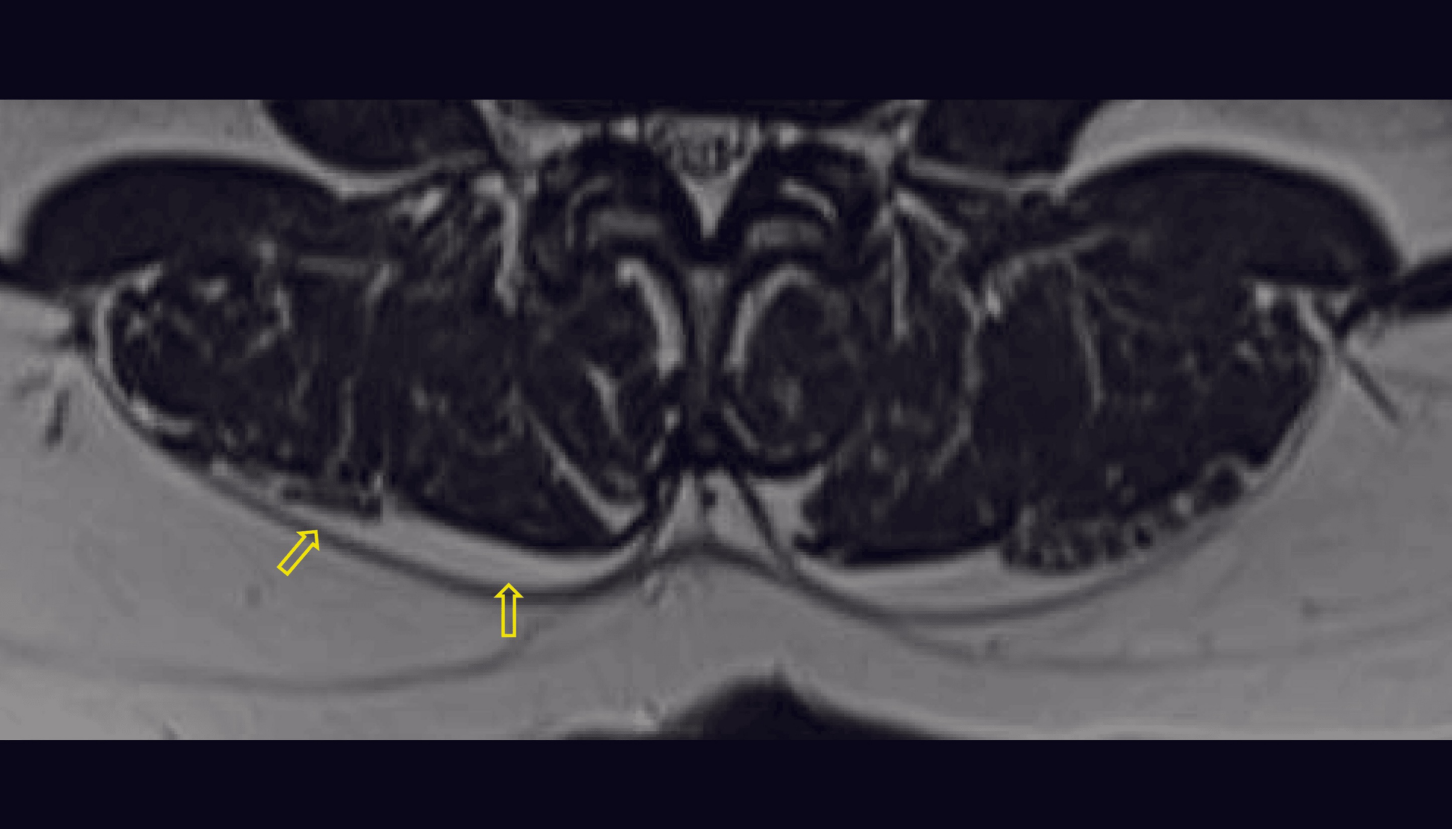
Axial T2-weighted MR image with yellow arrows pointing the epimuscular fat deposition along the posterior border of paraspinal muscles, with a score of 2 MR: magnetic resonance
